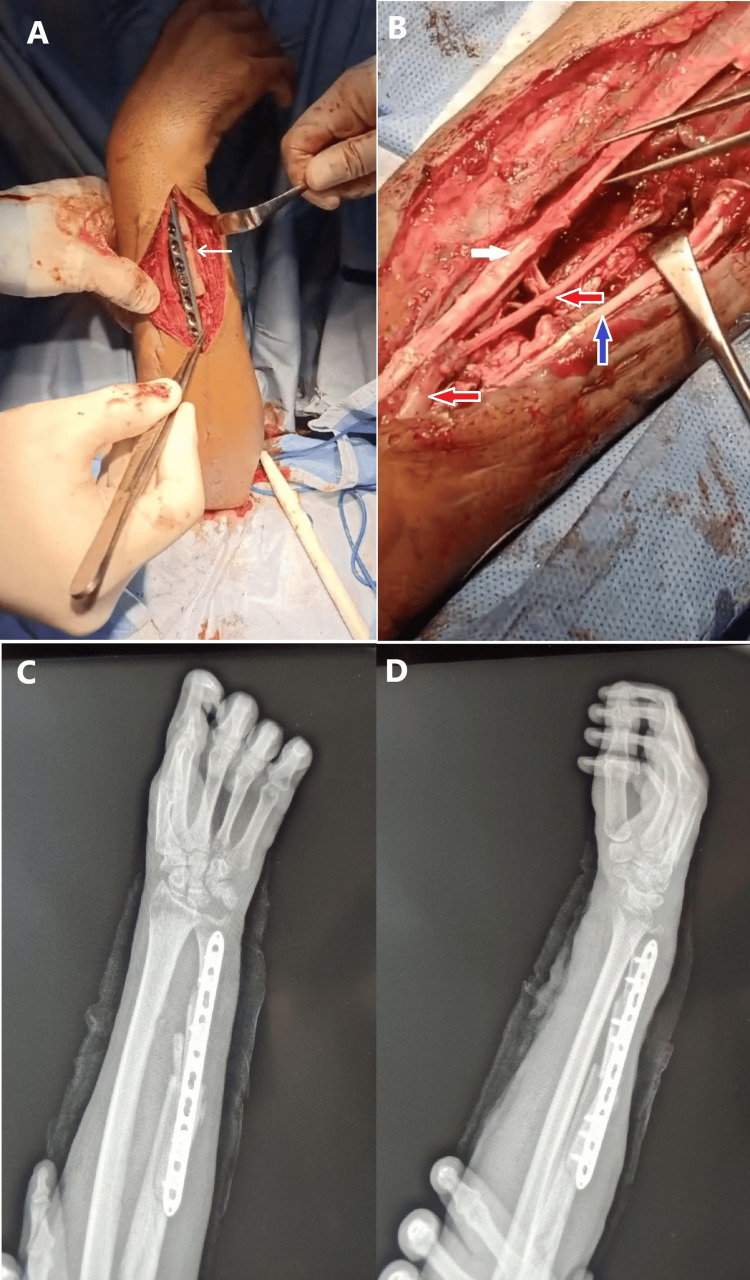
(A) Intraoperative image showing ulnar fixation using a dorsal locking compression plate (LCP) with the gap filled using a fibular graft. The ulnar nerve is visible in the surgical field (arrow). (B) Intraoperative image after exploration of the volar forearm wound, revealing a 7 cm gap in the median nerve and injuries to the flexor tendons (FDS, FDP, FI). The median nerve was reconstructed using cutaneous nerves harvested from the forearm (red arrow), while the flexor tendon gaps were bridged using half of the FCR tendon (blue arrow); the white arrow indicates the FCR. (C, D) Postoperative forearm X-rays show ulnar fixation with the fibular graft in place. The longest available LCP was utilized, though it did not span the proximal fracture, which was confirmed to be healed both clinically and radiologically during surgery. FDS: flexor digitorum superficialis; FDP: flexor digitorum profundus; FI: flexor indicis; FCR: flexor carpi radialis
